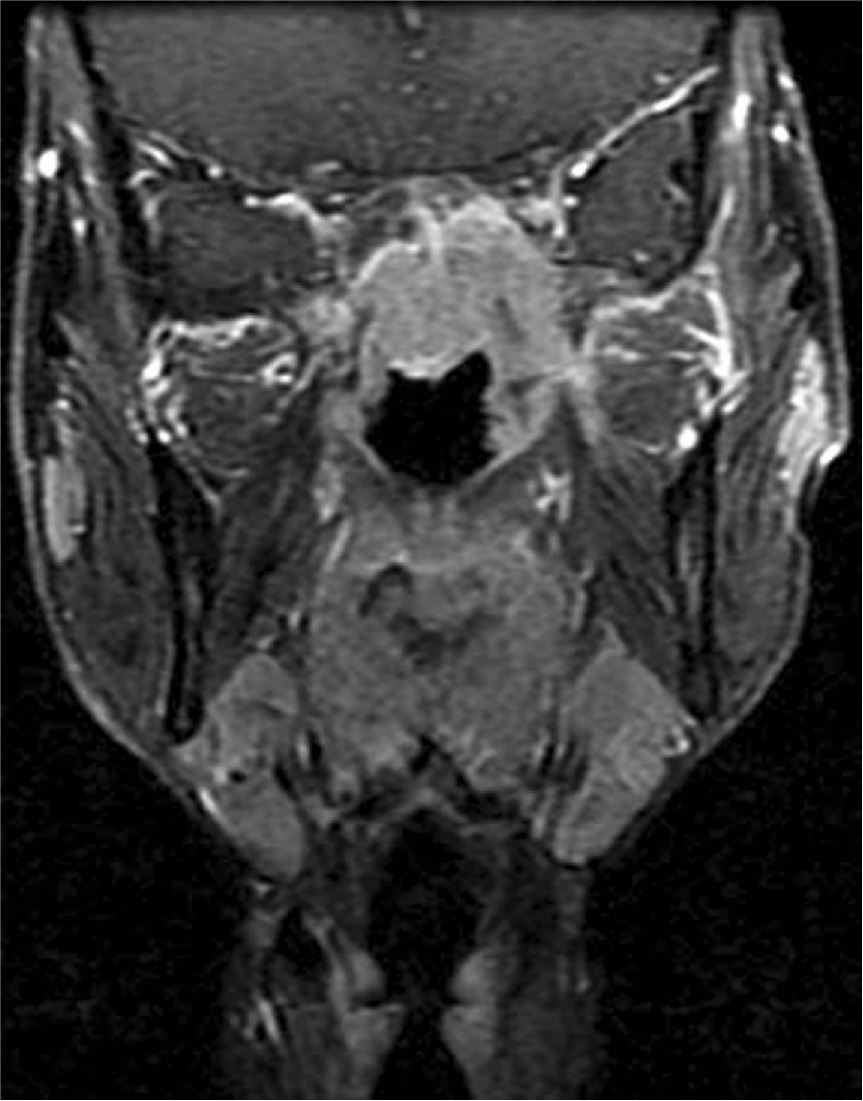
Image of tumor invasion into the sphenoid sinus in patient with NPC. A contrast enhanced coronal T1-weighted MR image revealed that the mass connected with the primary nasopharyngeal lesion invaded into the sphenoid sinus and that the floor of the sphenoid sinus was destroyed.
